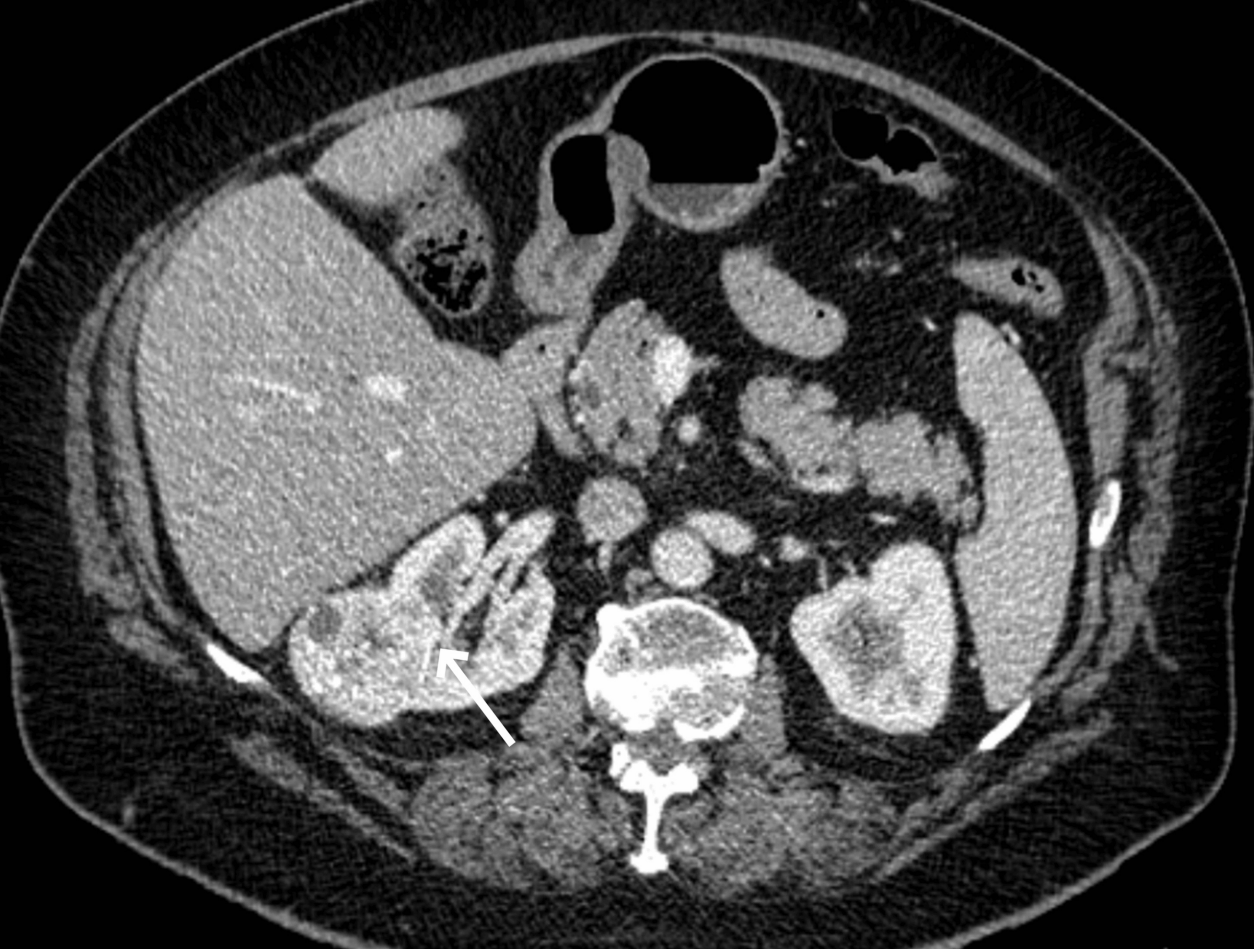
Contrast-enhanced, axial CT image. A hypervascular, partially exophytic right renal tumor. The deepest part of the tumor (arrow) is abutting the renal pelvis.
